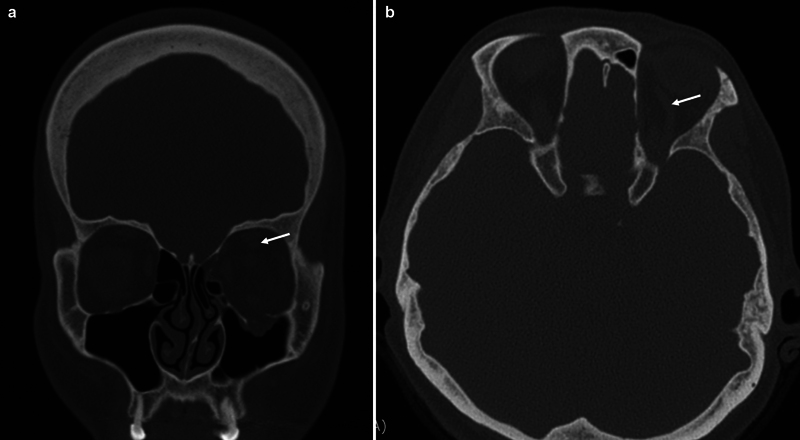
Noncontrast CT of the patient's first hospital visit. Superior ophthalmic vein dilatation is confirmed. (
